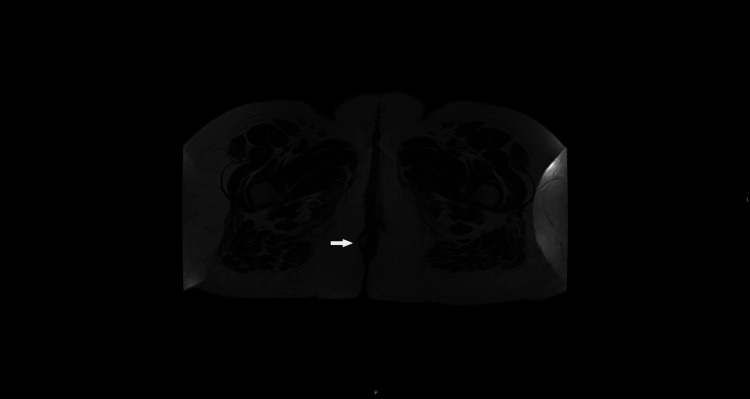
T2-weighted MRI scan of the pelvis axial section with an arrow demonstrating a small lesion in the perianal area
